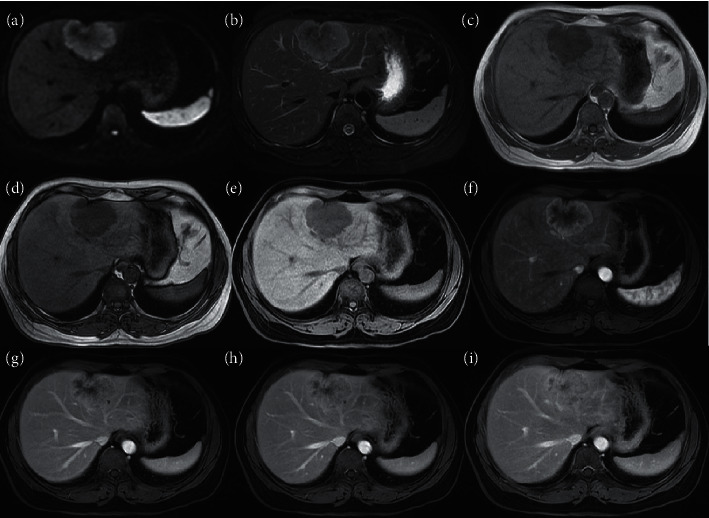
MR images in a 48-year-old HBV-negative woman with iCCA confirmed by surgical resection. (a) DWI image; (b) T2WI; (c) T1WI image in-phase; (d) T1WI image out-phase; and 3D fat-suppressed T1WI precontrast (e) and in the (f) arterial, (g) portal phase, and (h, i) delayed phases. The tumor measures 49 × 65 mm, retracts the liver surface, and shows targetoid appearance on DWI, rim APHE, delayed central enhancement, and peripheral washout appearance.
